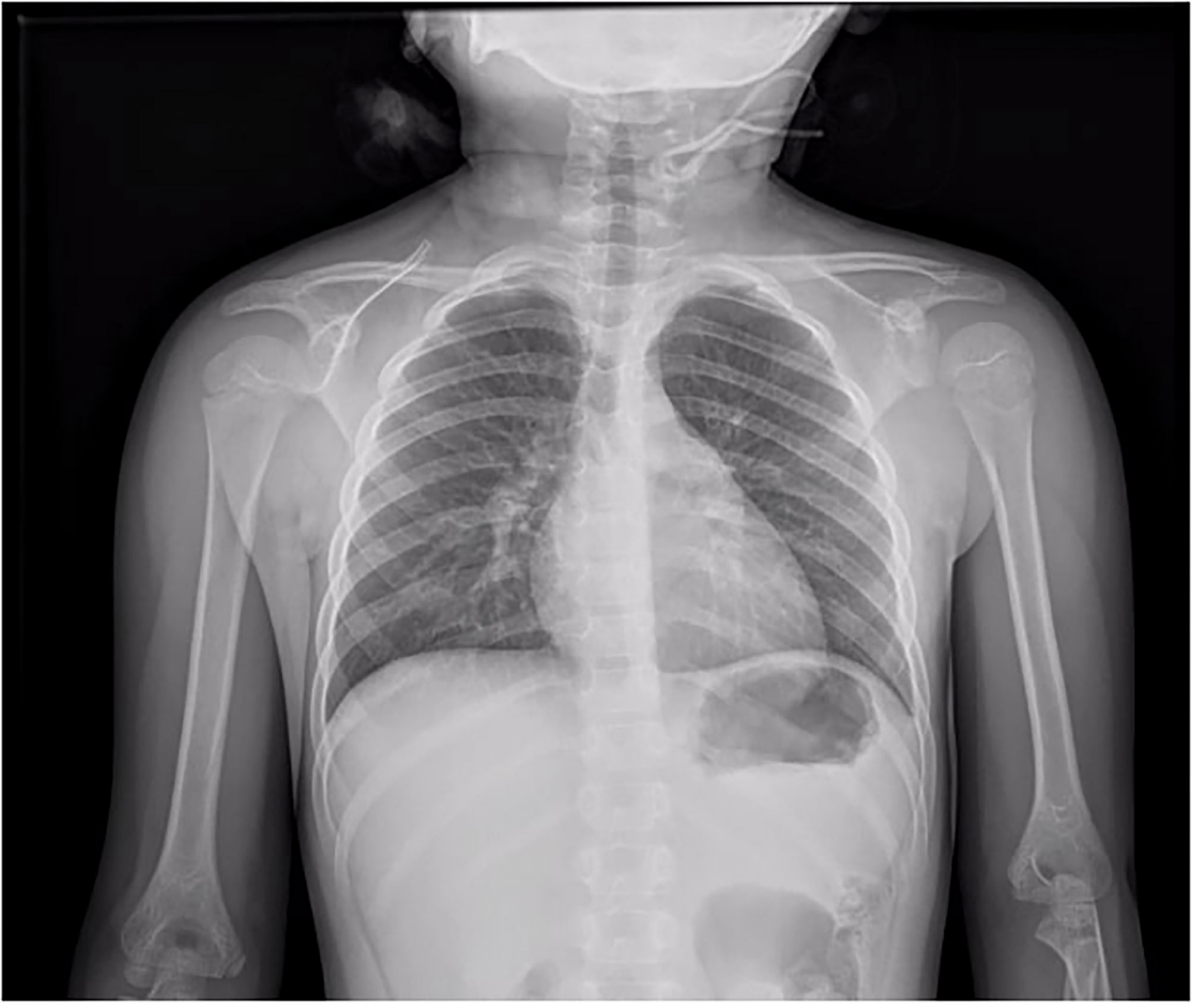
Chest x-ray showed slight thickening of the interstitial web with mild bilateral pleural effusion.
